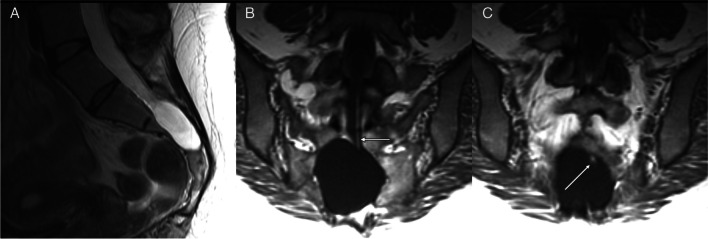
A T2-weighted sagittal image of a meningeal diverticulum remodelling the sacral canal. B T1-weighted coronal image of the sacrum demonstrating a subtle lipoma of the filum terminal (arrow) associated with the meningeal diverticulum. C The fatty filum traverses the neck and merges before merging with the wall of the diverticulum (not shown)
